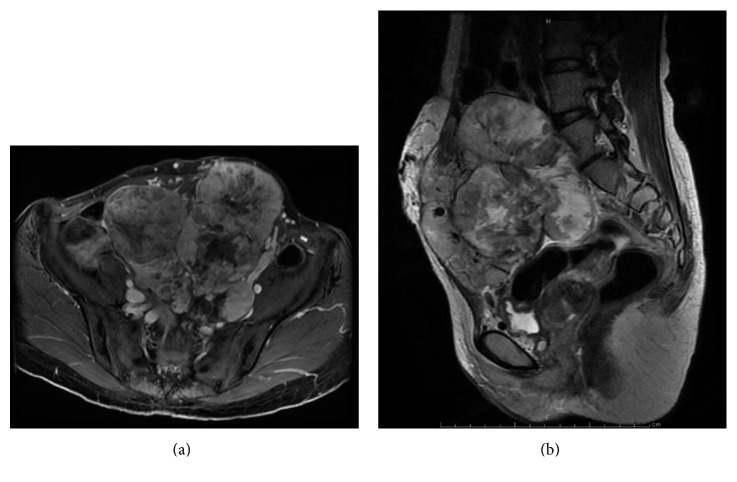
Magnetic resonance imaging findings at the initial visit. (a) T1-weighted fat suppression gadolinium-enhanced image (axial view). (b) T1-weighted fat suppression gadolinium-enhanced image (sagittal view). This MRI showed a large lobulated lesion with strong enhancement. The tumor was located at the abdominal wall, bulging to the lumbar spine.
